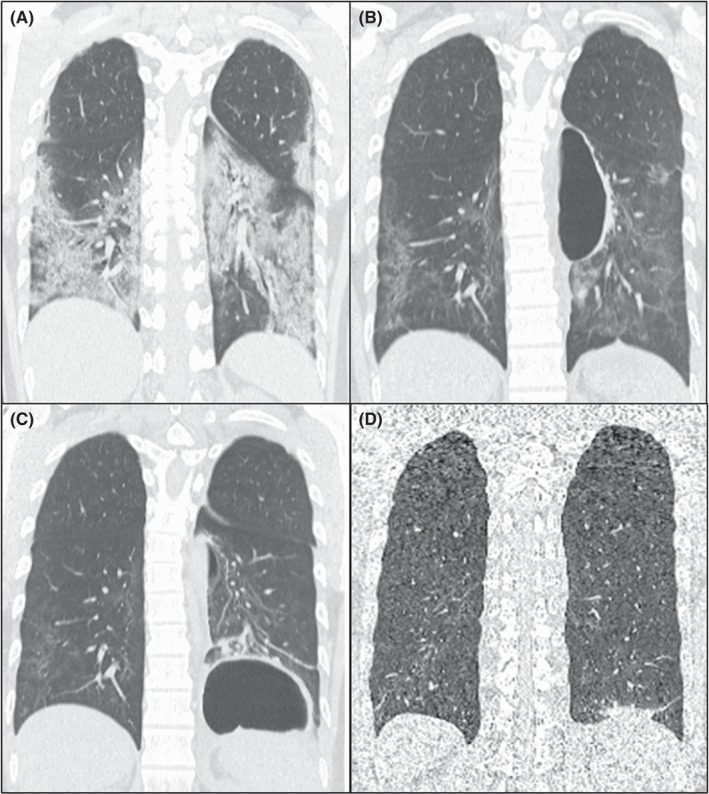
(A) Coronal computed tomography (CT) on admission demonstrated bilateral patchy consolidation and ground‐glass opacities (GGO). (B) Coronal CT on discharge revealed a cystic feature in the left mediastinal side. Bilateral consolidation and GGO were attenuated. (C) Coronal CT after four weeks from discharge demonstrated an additional cystic feature in the left epiphrenic side. (D) Coronal CT after eight weeks from discharge revealed disappearance of the cysts, bilateral consolidation, and GGO
